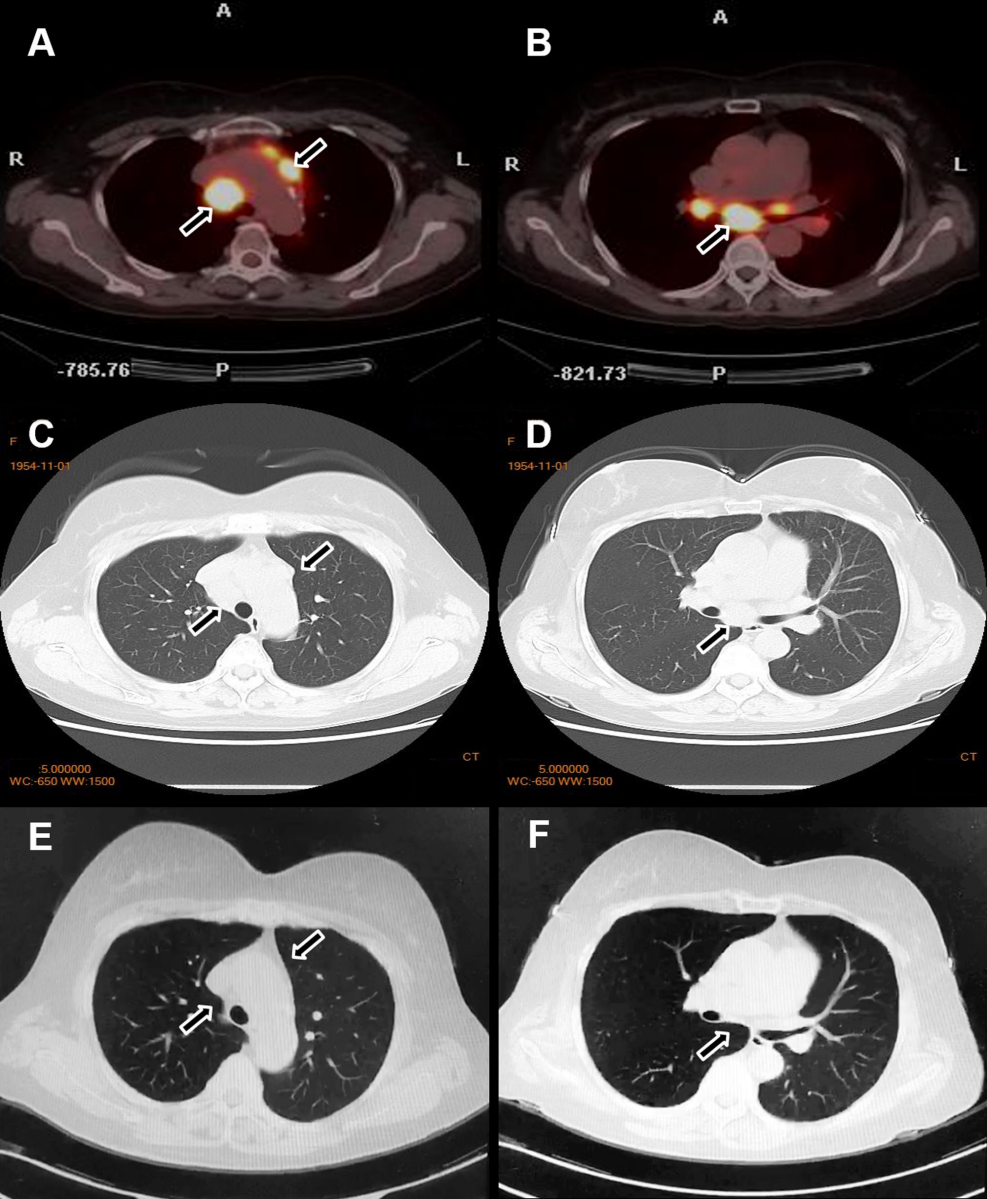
Changes in the lymph nodes before and after treatment. Before treatment, PET-CT (A, B) and chest CT (C, D) revealed enlarged lymph nodes in the bilateral supraclavicular and hilar regions. After treatment, a marked reduction in the size of the lymph nodes was observed (E, F).
